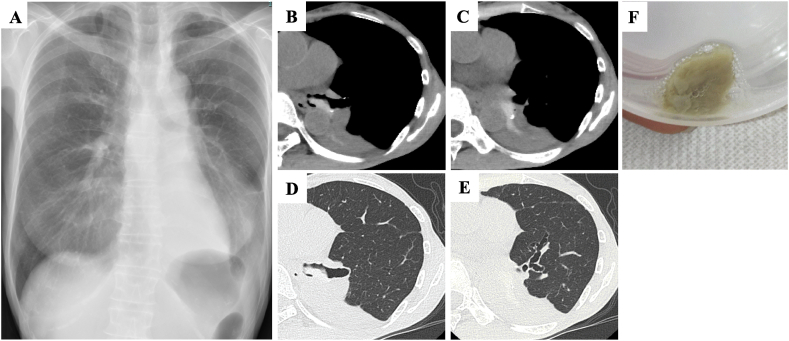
Chest imaging findings on admission. Chest radiograph showing the collapse of the left lower lung (A). High-resolution CT showing high-attenuation mucus (HAM) in the lower lobe bronchus (B, C) and left lobe atelectasis (D, E). Mucus plugs in the sputum (F).
